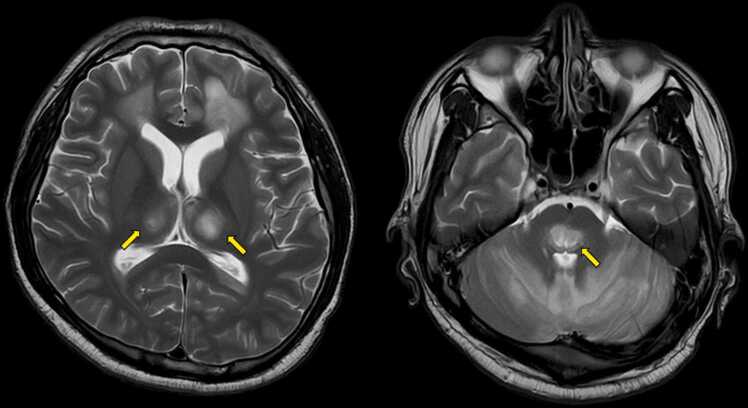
Brain MRI on T2 sequence showing hyperintensities in bilateral thalamus and pons on T2.
